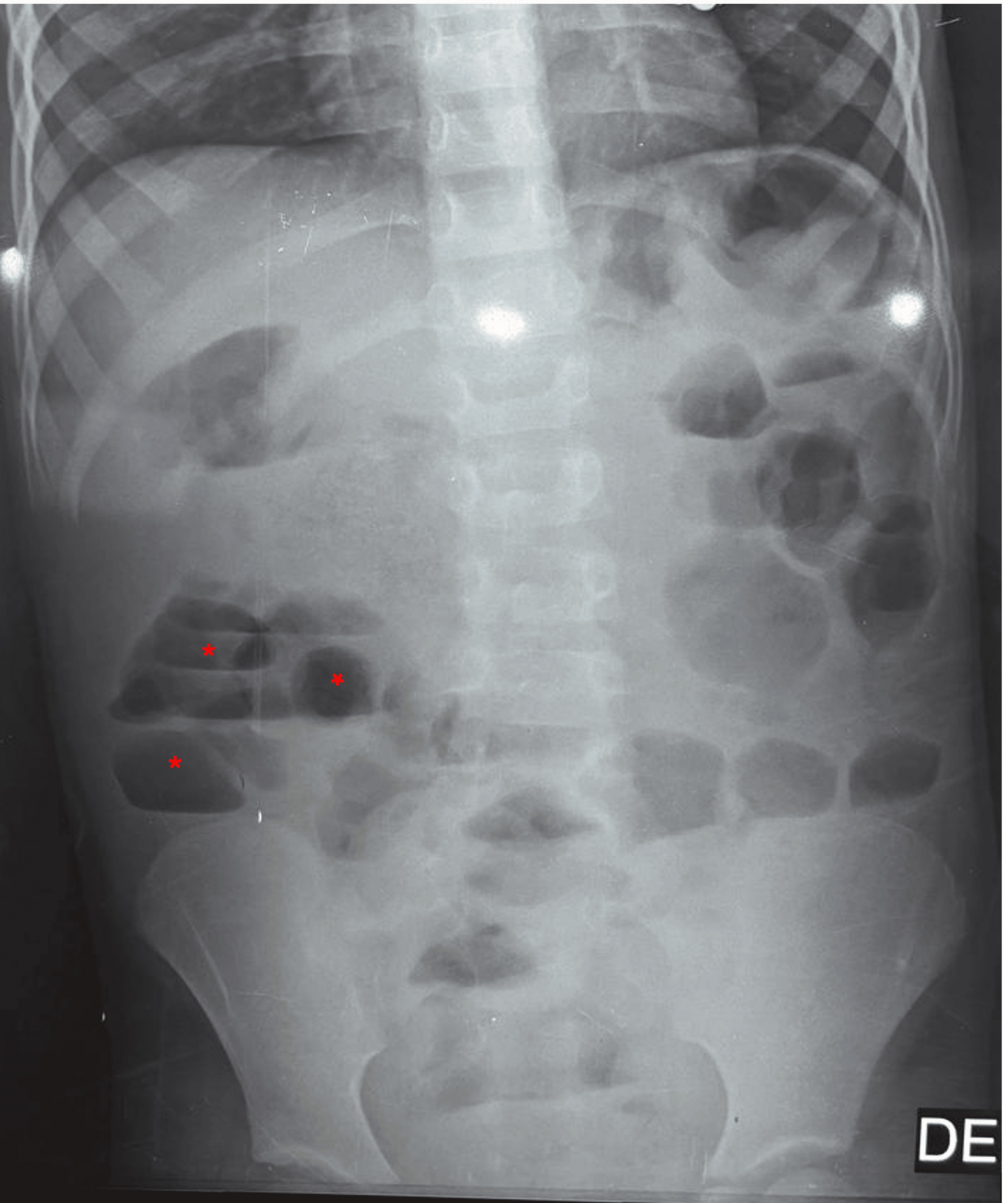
Upright abdominal X-ray showing small bowel air-fluid levels (red star)
